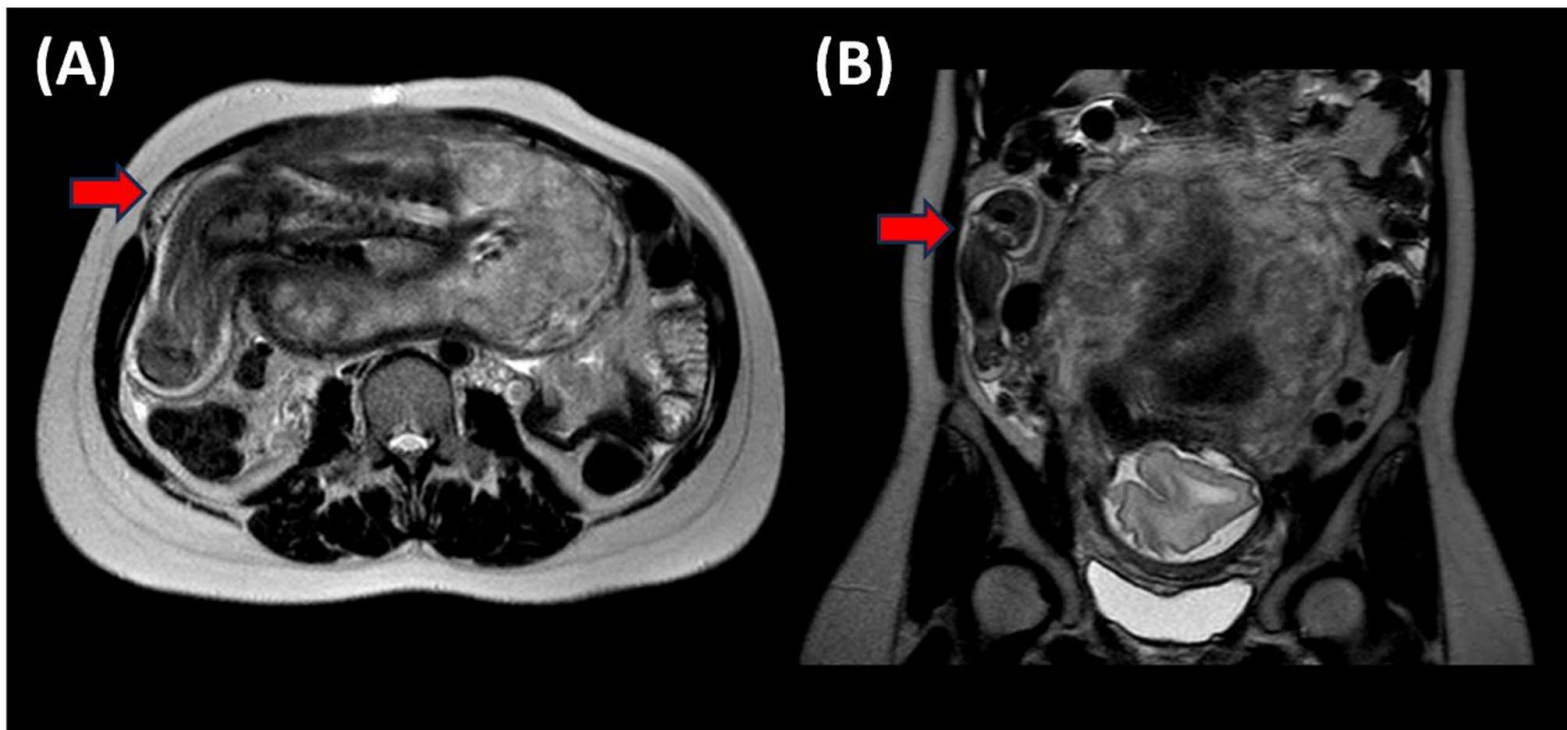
MRI confirmation of uterine rupture. A Axial MRI image demonstrating uterine rupture at the right interstitial region with fetal parts herniating through the uterine wall (arrow indicates the site of rupture). B Sagittal MRI image showing the uterine rupture and protrusion of fetal parts (arrow indicates the area of uterine wall defect and herniation)
